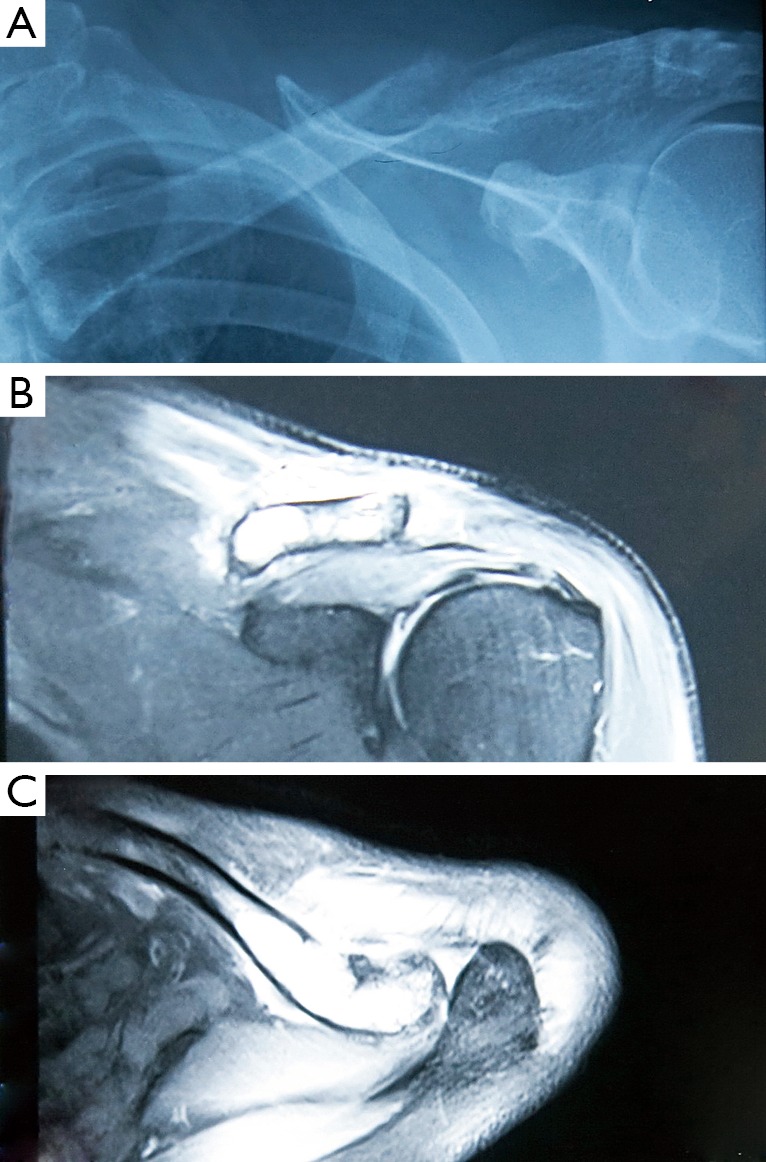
X-ray and MRI results of a 64-year-old patient. (A) X-ray result displayed an osteolytic lesion in the lateral third of left clavicle. (B,C) T2-weight MRI to the left clavicle revealed high-intensity lesions.
